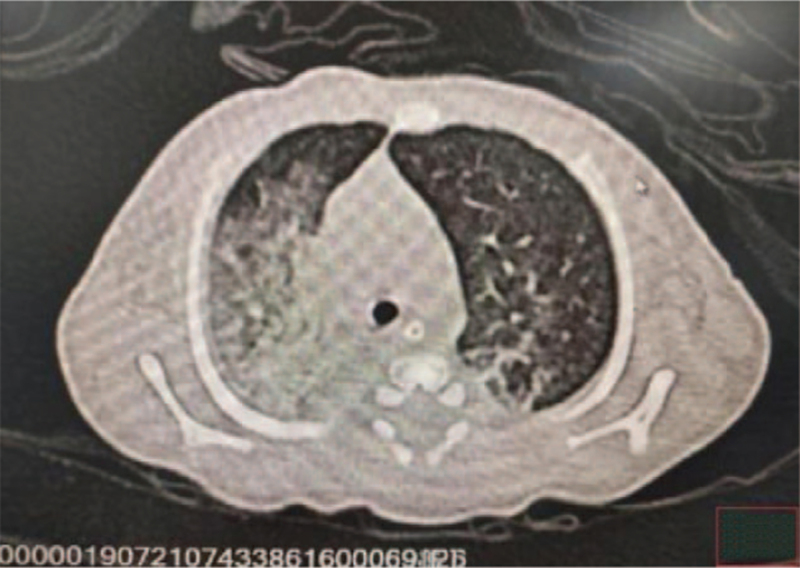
The CT showed no abnormality about bronchus and esophagus.
